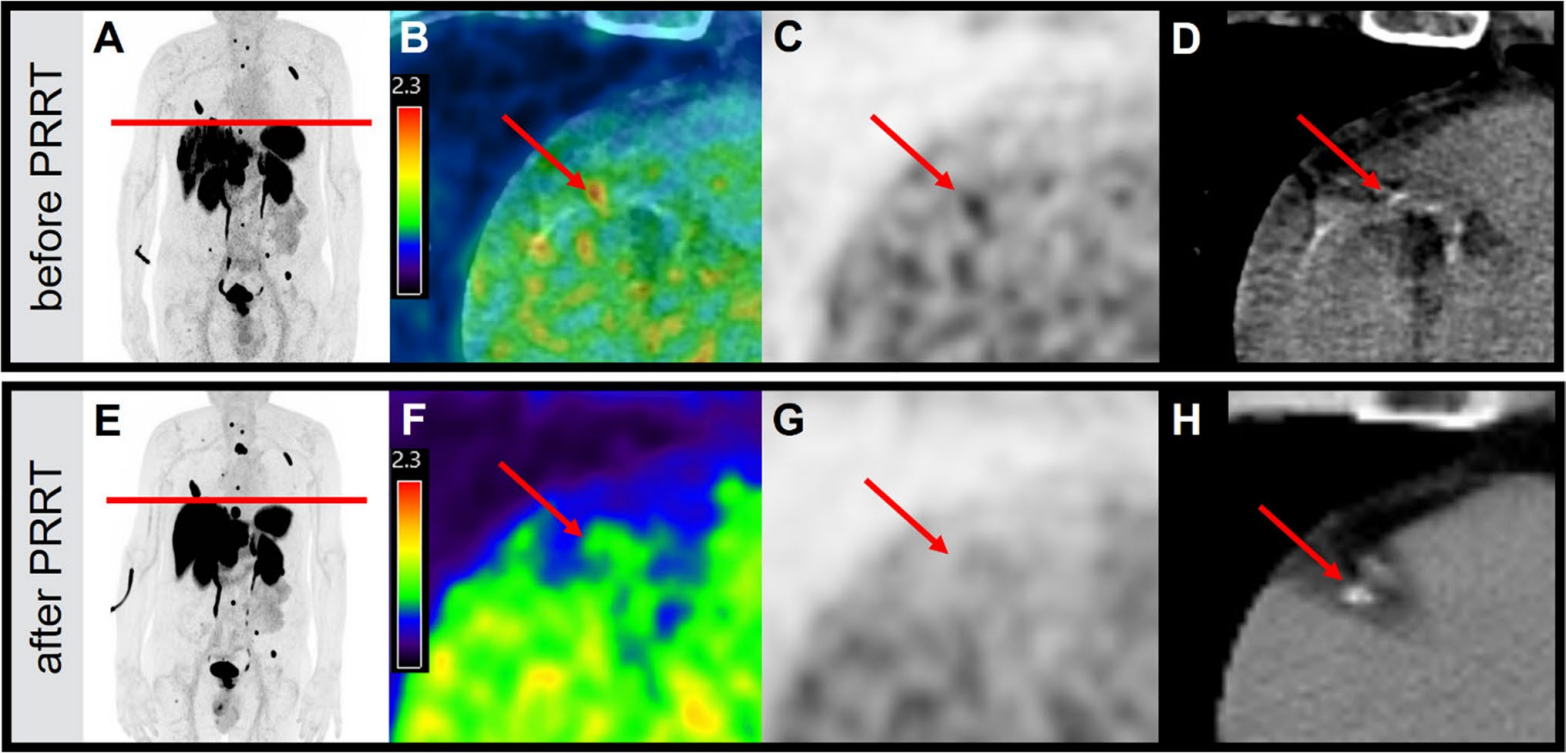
[^68^ Ga]Ga-DOTA-TOC uptake before and after PRRT. A 80 y/o male patient with metastatic neuroendocrine tumor of the pancreas was treated with peptide receptor radionuclide therapy (PRRT). Shown are maximum intensity projections (MIP – A/E), axial fusion images (B/F), stand alone PET (C/G) and stand-alone CT (D/H) images of a calcified inflamed RCA plaque (marked with a red arrow). A–D show the [^68^ Ga]Ga-DOTA-TOC uptake before and E–H after PRRT
